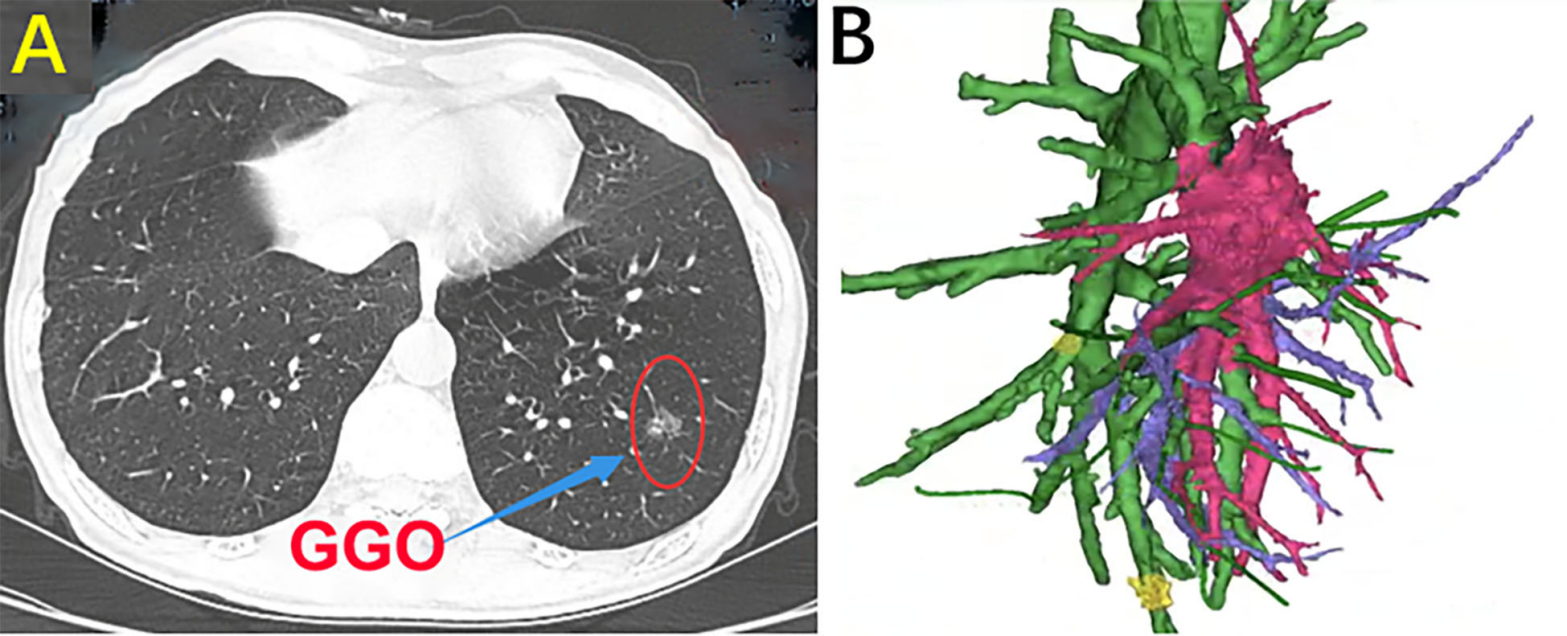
(A) Chest CT scan. (B) Three-dimensional computed tomography-bronchography and angiography (3D-CTBA).
